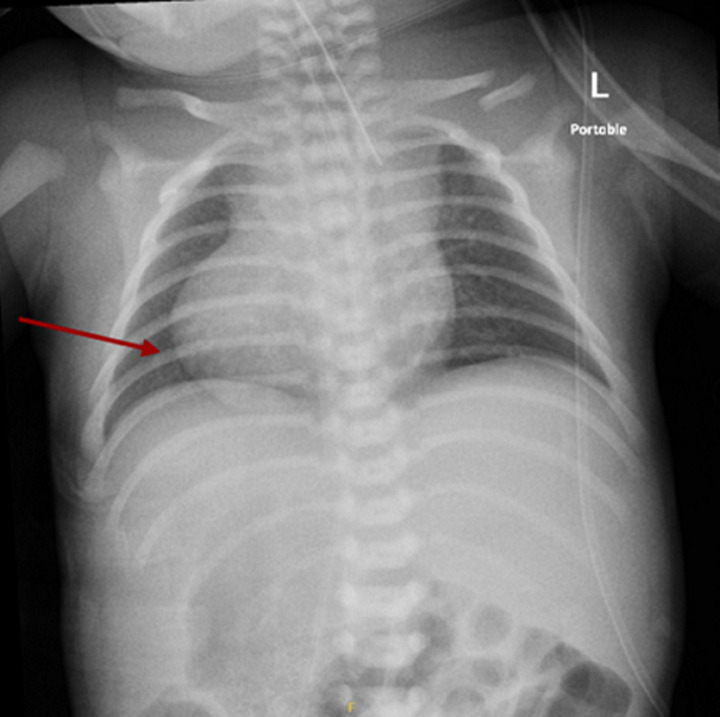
initial chest X-ray showed situs inversus, and clear lung fields; the red arrow points to dextrocardia; normal cardiothoracic ratio
